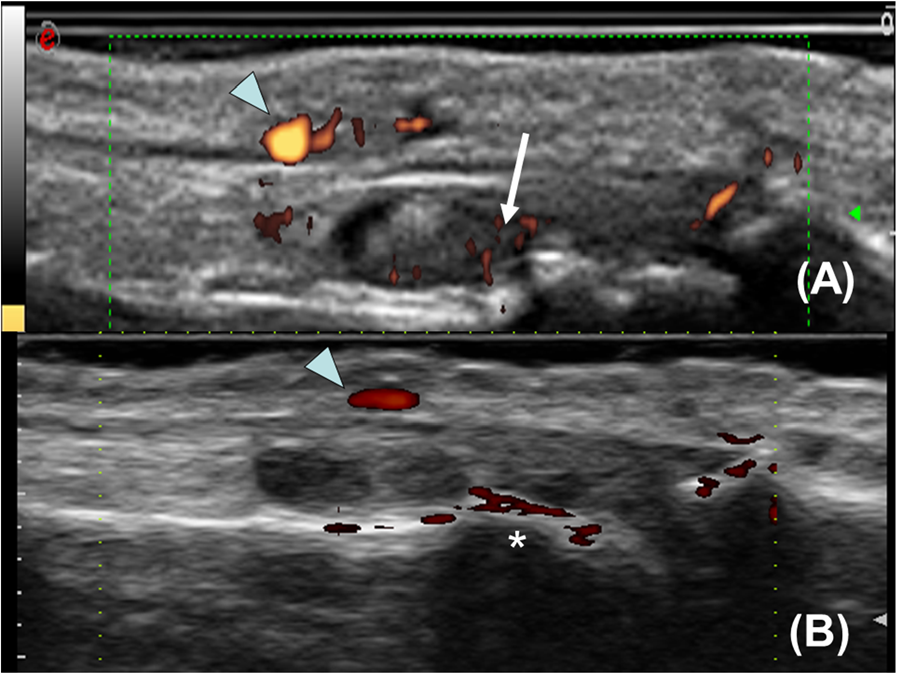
Different performance of the power Doppler ultrasound modality of two machines in one patient. (A) Machine B, presence of a positive power Doppler signal (arrow) within the region of synovial proliferation. (B) Machine A, this signal is absent. Arrowhead, vessel; *noise on cortical surface.
